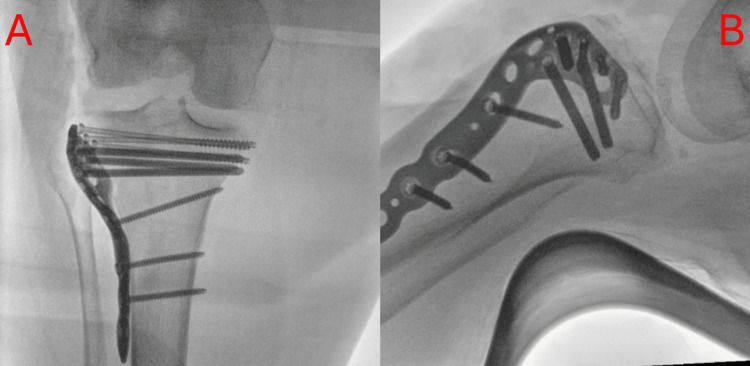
Intraoperative anterior to posterior (a) and lateral (b) X-rays of tibial plateau fixation A) Plain intraoperative anterior to posterior radiograph of the patient's right knee. This image shows lateral plate and screw fixation of the tibial plateau fracture; B) Plain lateral intraoperative radiograph of the patient's right knee. This image shows the lateral plate, with screw fixation, of the tibial plateau fracture.
